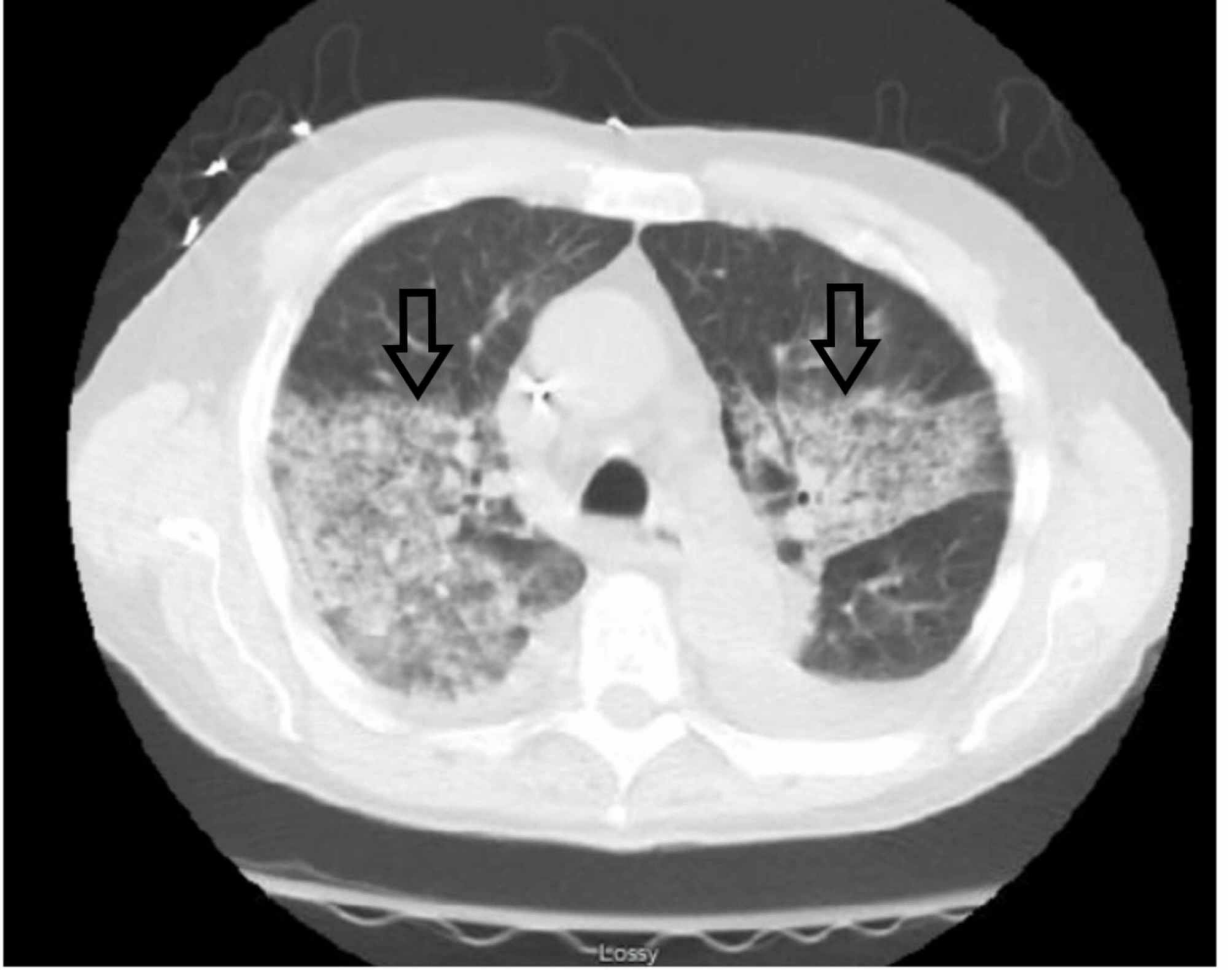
Computed tomography scan of the chest without contrast demonstrating bilateral perihilar heterogeneous opacities
